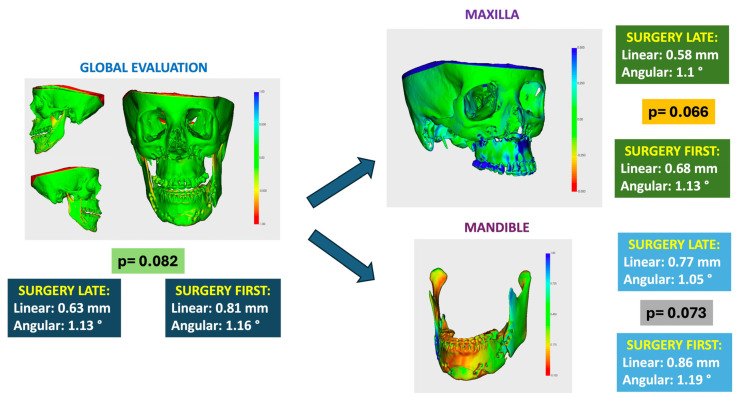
Outcome analysis of surgical precision through comparison between VSP and postoperative CBCT.
